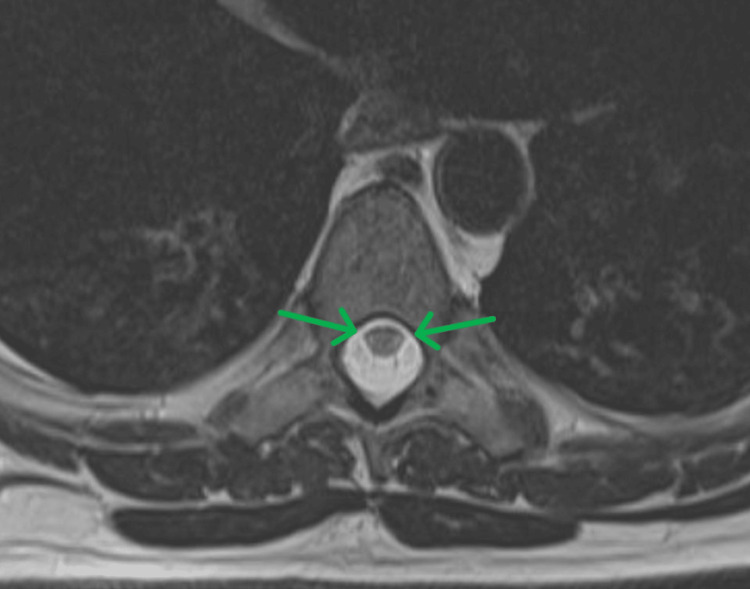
MRI thoracic spine revealing subtle T2 hyperintensity of the lateral columns
